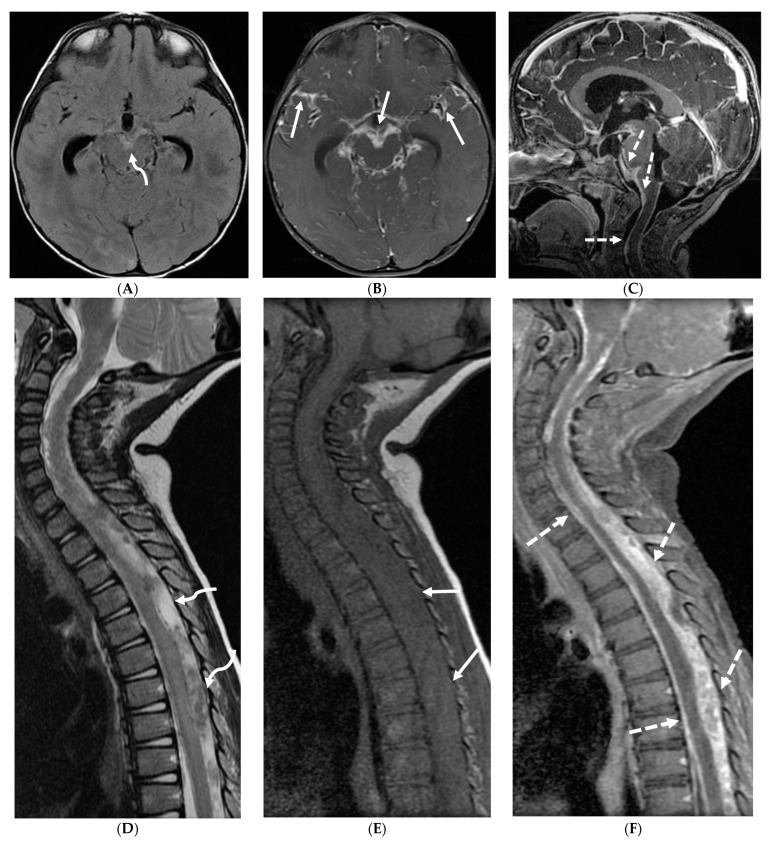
3-year-old boy with 2 weeks history of headache and vomiting. Axial FLAIR (A), post contrast axial T1 FS (B) and sagittal 3D inversion recovery (C) images demonstrate FLAIR hyperintensity in the interpedencular cistern (curved arrow) and mild hydrocephalus. Extensive meningeal enhancement most prominent at the skull base, basal cisterns, and Sylvain fissures (arrows), but extending throughout the brain. There is meningeal enhancement, with coating of the brainstem extends inferiorly along the cervical spinal cord (dashed arrows). Sagittal T2 (D), sagittal T1 (E) and fat saturated T1 post (F) images show extensive leptomeningeal with predominantly solid and some cystic nodules (curved arrows) on T2 sequence and isointense on T1 (arrows). Lesions predominantly involve the posterior spinal canal, causing mass effect and anterior displacement of the spinal cord. The solid nodules show enhancement after contrast injection and extensive uniform diffuse LME around the cord (dashed arrows). Pathology: Primary Meningeal Rhabdomyosarcoma.
